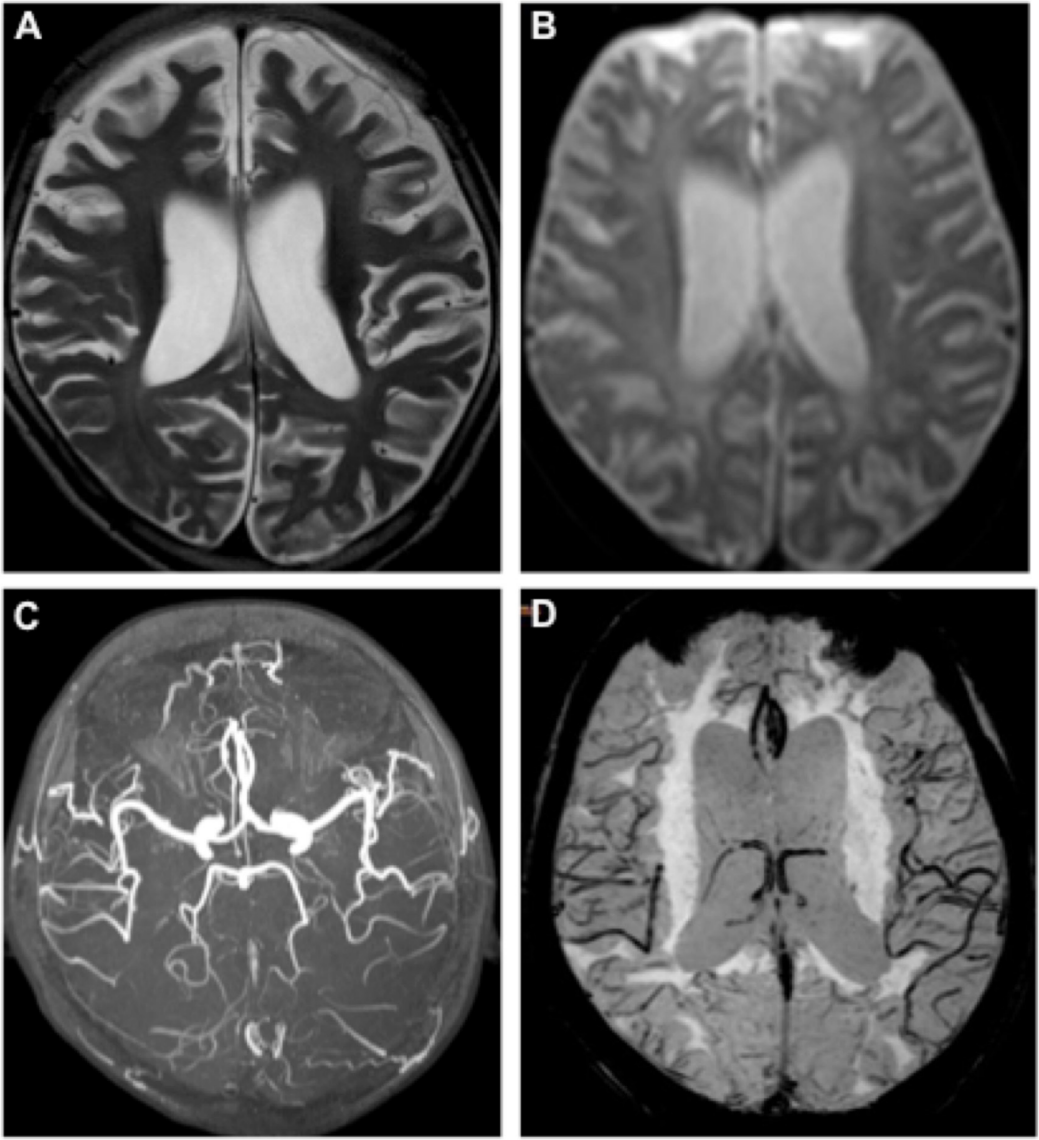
MR images of the RTT patient Axial T2-weighted image (A) showed diffuse high intensity areas in the posterior periventricular white matter without obviously worsen. DWI (B), MRA (C), and SWAN (D) at age of 8 were normal.
